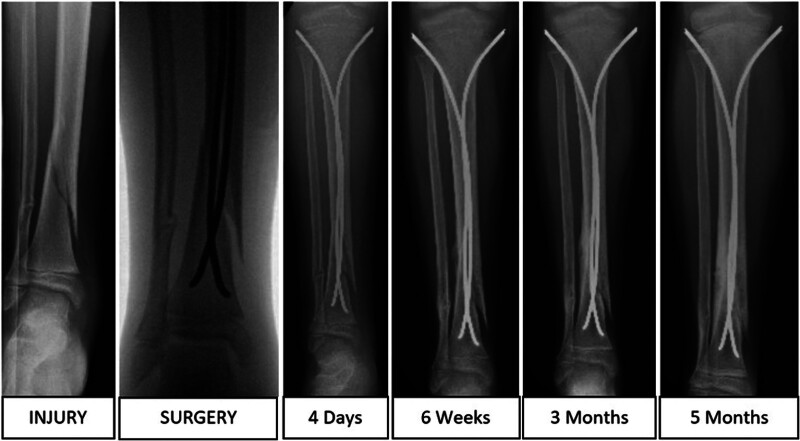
Anteroposterior radiographs of 6-year-old female patients with diametaphyseal fracture of right distal tibia with 6° of valgus on final radiograph prior to implant removal.
